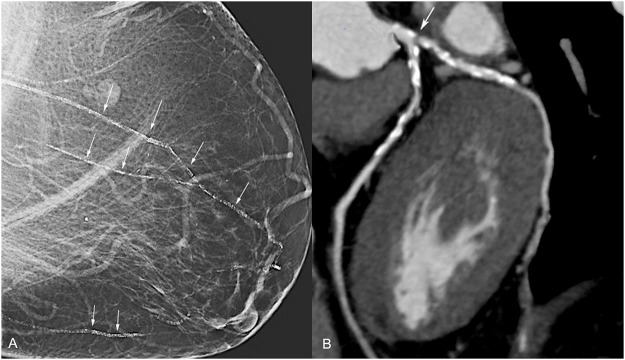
Images from a patient with mammography and CT angiography findings. Screening mammography in 65-year-old patient demonstrates severe vascular calcifications (Grade 4) on screening mammogram (A). Corresponding coronary CT angiogram (B) shows diffusely calcified proximal left anterior descending and left circumflex artery with focal high-grade stenosis of (arrow).
